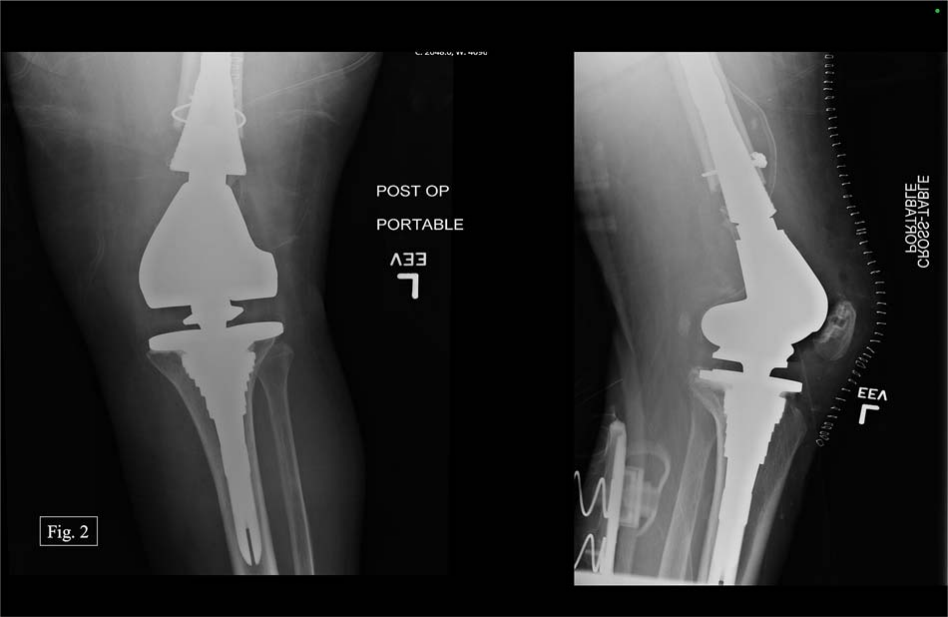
Left, immediate postoperative AP and lateral X-rays.
